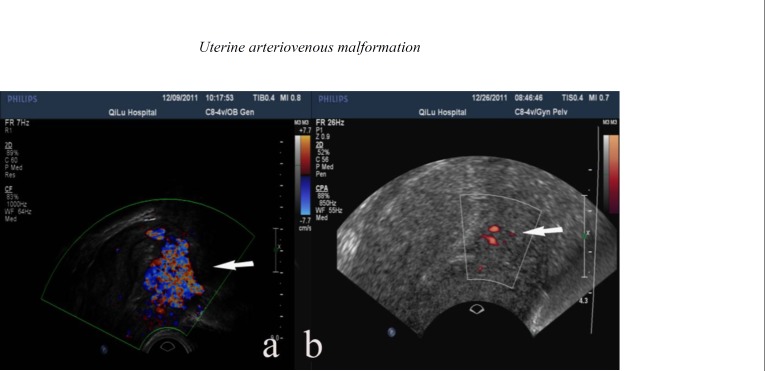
Color Doppler imaging features of uterine arteriovenous malformation (AVM) pre- and post- embolization. (a) Color Doppler image showing mosaic pattern and turbulent flow (arrow) pre-embolization. (b) Color Doppler image two weeks after embolization indicating markedly reduced blood flow (arrow
